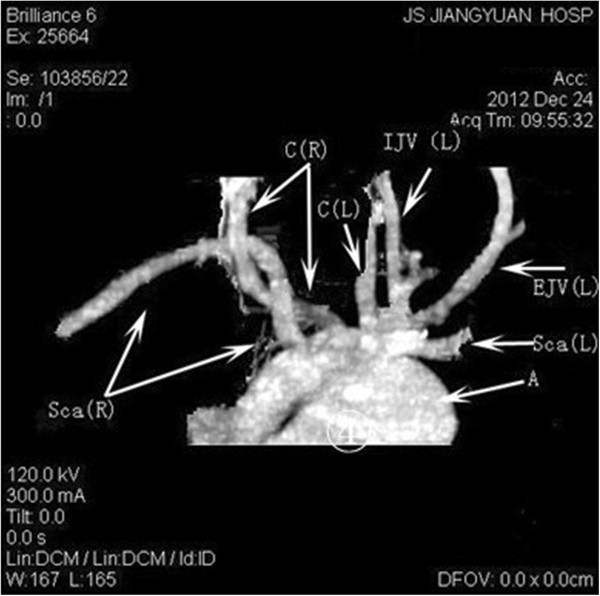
Computed tomographic angiography showing the absence of the brachiocephalic artery; the right subclavian artery and right common carotid artery originate from the aortic arch. Sca(L), left subclavian artery; Tr, trachea; Sca(R), right subclavian artery; C(R), right common carotid artery; C(L), left common carotid artery; A, aortic arch; Ep, esophagus.
